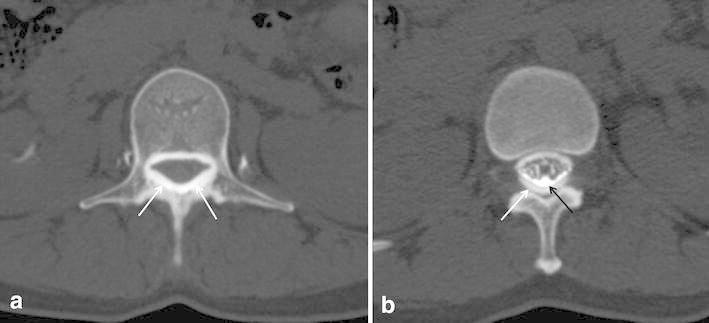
A 37-year-old woman with spontaneous intracranial hypotension (case #2). Initial myelography failed due to extensive subdural contrast injection. Axial MDCT-myelography (a) revealed a dense high-attenuated subdural area (*white arrow*) with dark attenuated dural sac. So, blind epidural blood patch was done in the C7/T1 level, but the symptom did not improve after that. One week later MDCT-myelography was repeated. On axial CT image (b), contrast leakage was seen at the L1 level with “gray-rim sign” (peripheral leaked contrast with *white arrow* and central dense contrast with *black arrow*). Targeted epidural blood patch was done at the L1 level; the symptoms of the patient relieved dramatically, and she was discharged the next day
